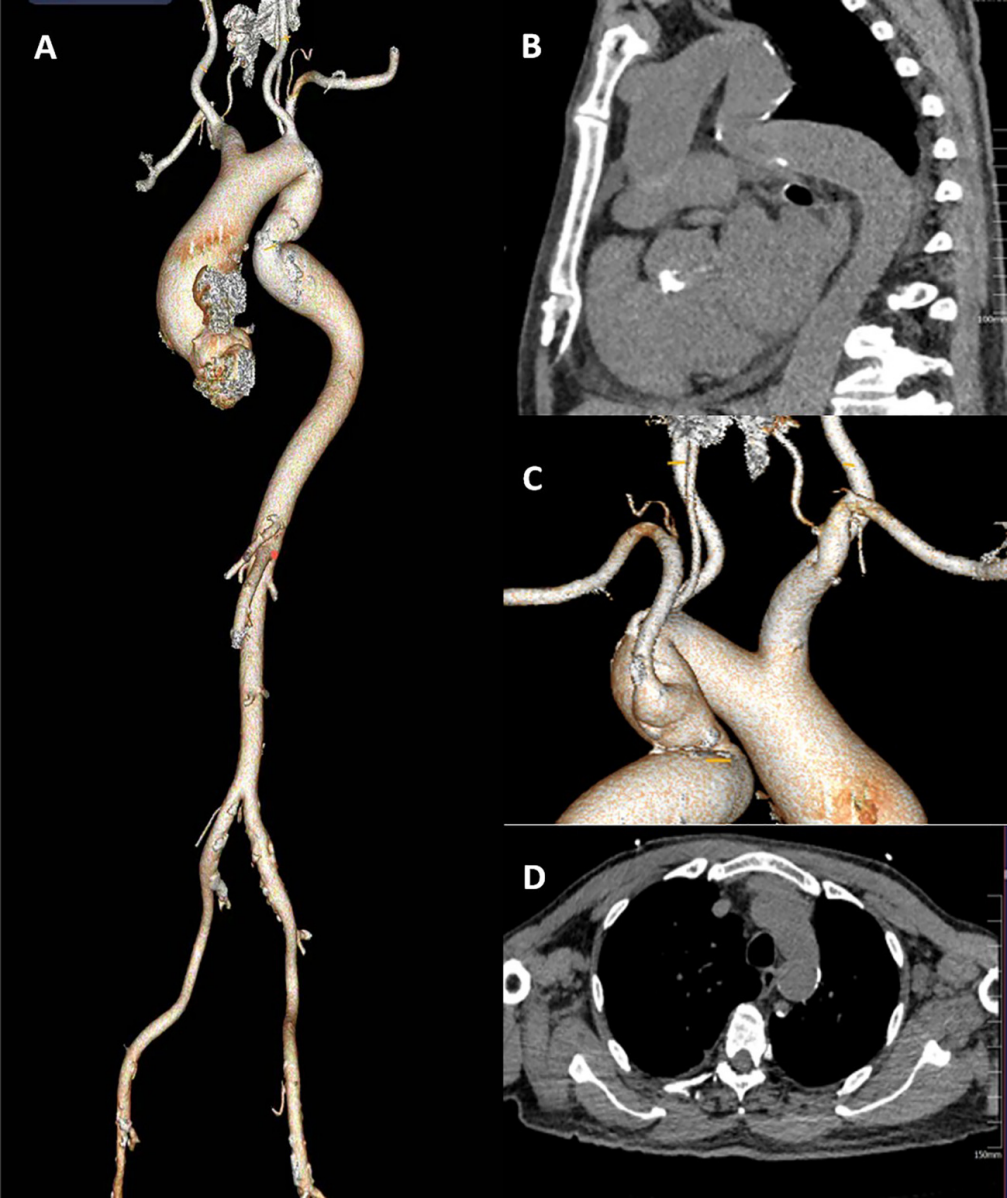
(A) the full CT of the aorta. (B) a Z-shaped fold tortuosity in the descending aortic arch.(C) the left common carotid artery is free from stenosis or calcification, the Willis circle is complete. The right vertebral artery is dominant. (D) The narrowest segment of the descending aorta).
